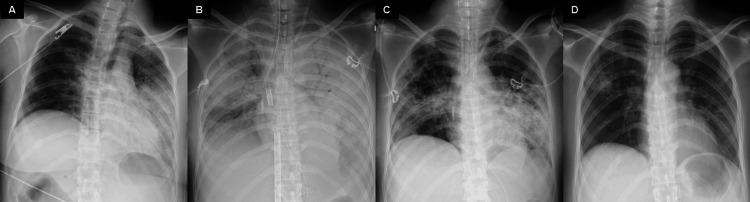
Changes in chest X-ray findings The lung consolidation, initially confined primarily to the left side at admission (A), had significantly worsened bilaterally by the time ECMO was initiated (B). By the time the patient was weaned off ECMO, consolidation in the bilateral lung fields had significantly improved (D), with residual consolidation limited to the right middle and left middle and lower lung fields. At discharge, after the patient had been weaned off the ventilator and no longer required oxygen, the chest X-ray findings were nearly normal (D). ECMO: extracorporeal membrane oxygenation
